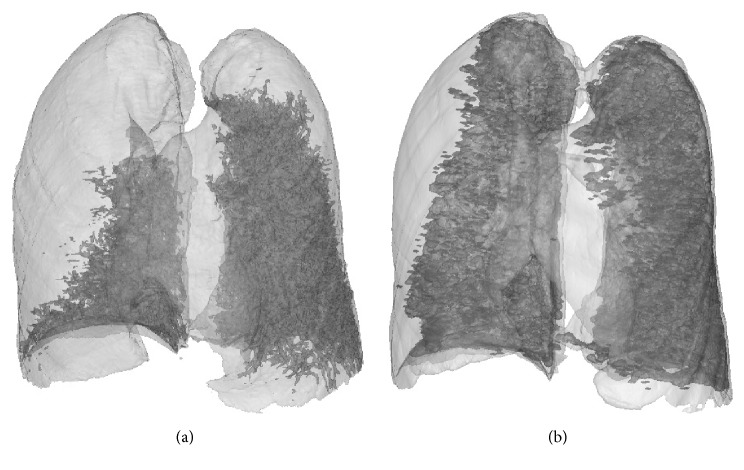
Injured lung images after 3D reconstruction at (a) 1st and (b) 2nd CT scans.
